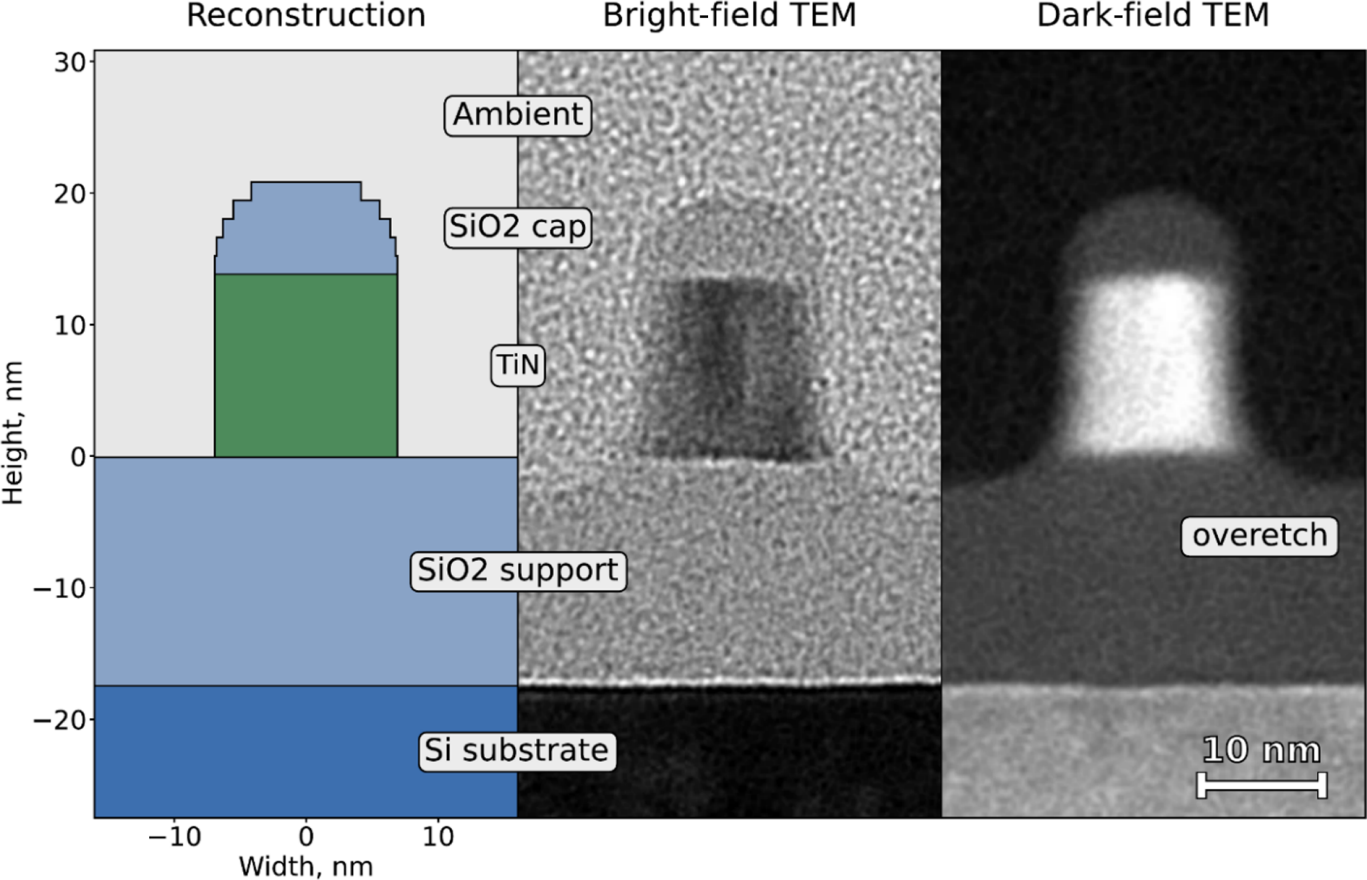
Final reconstructed profile of the nanograting based on the best fit of X-ray data in comparison with TEM images. Most of the features besides overetch are matched.
